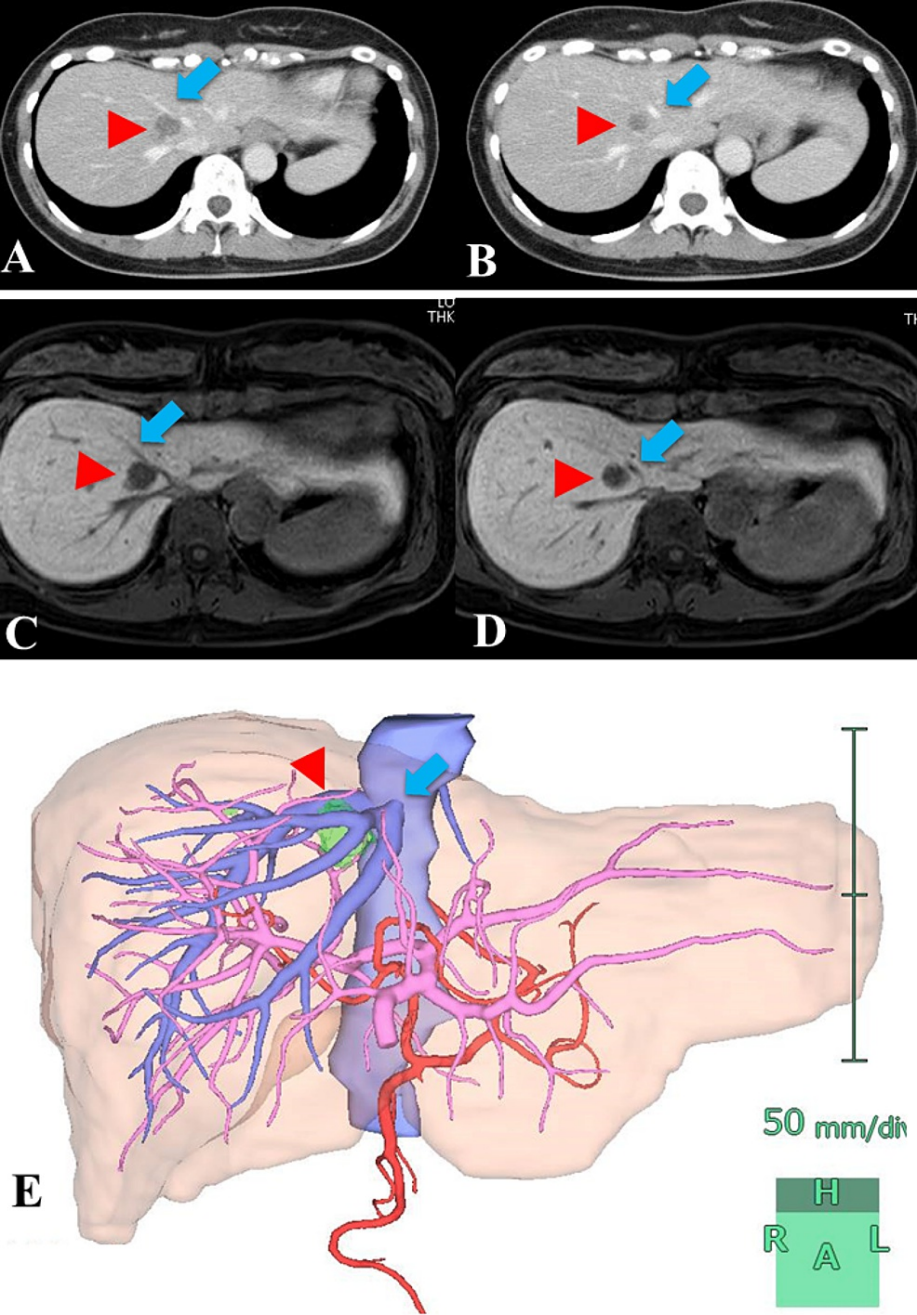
Preoperative image in the current case (A) and (B) show contrast-enhanced CT findings. (C) and (D) show the Gd-EOB-DTPA MRI study. (E) shows the results of the 3D construction. (Red arrowheads indicate the tumor. Blue arrows indicate the middle hepatic vein.) The tumor with a maximum diameter of 20 mm in S8 was small, but the lesion was located at a site sandwiched between the RHV and the root of the MHV. CT: computed tomography, Gd-EOB-DTPA: gadolinium ethoxybenzyl-diethylenetriaminepentaacetic acid, MRI: magnetic resonance imaging, RHV: right hepatic vein, MHV: middle hepatic vein
